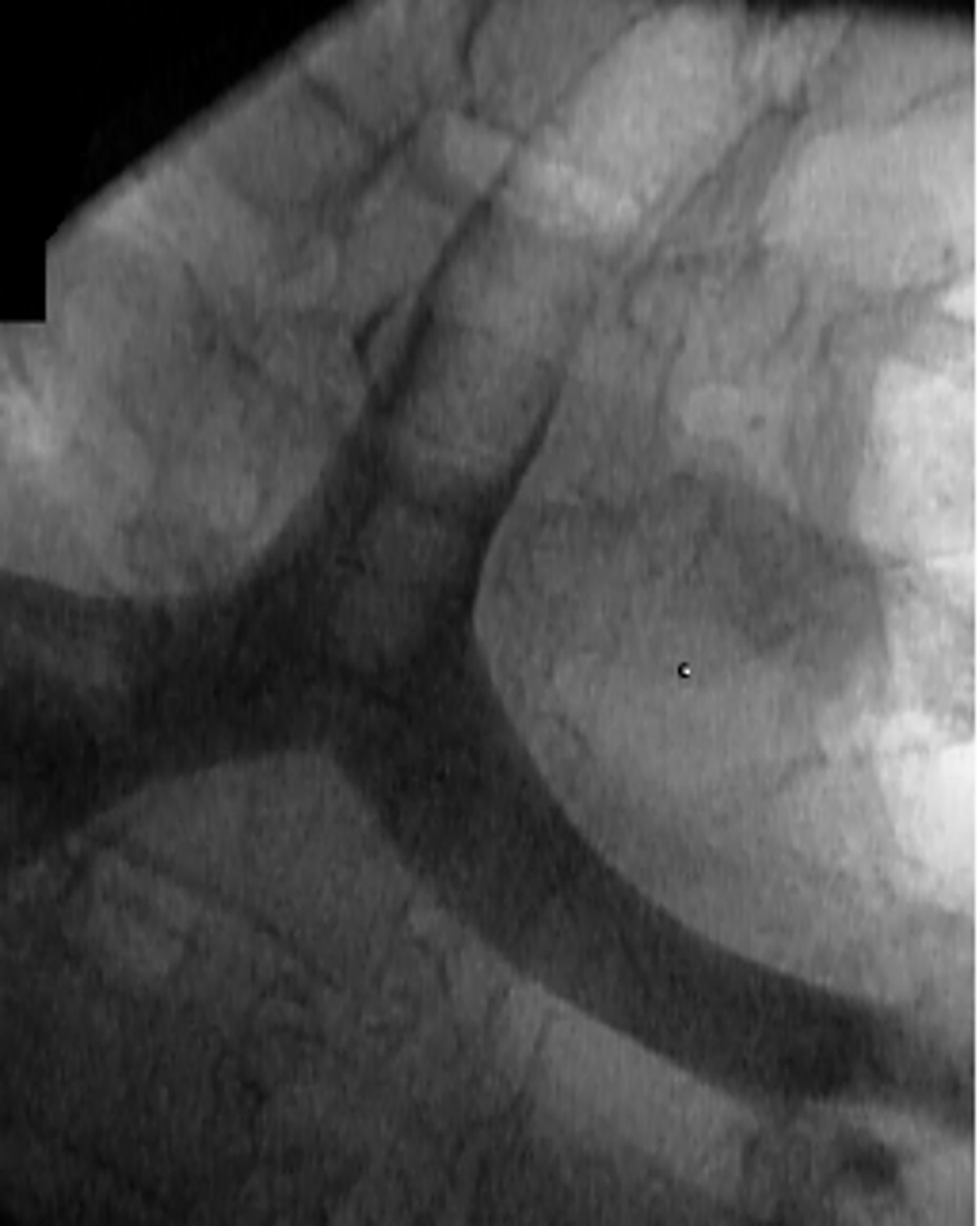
Pulmonary aspiration of barium sulfate radiopaque suspension, assessed by chest x-ray.
